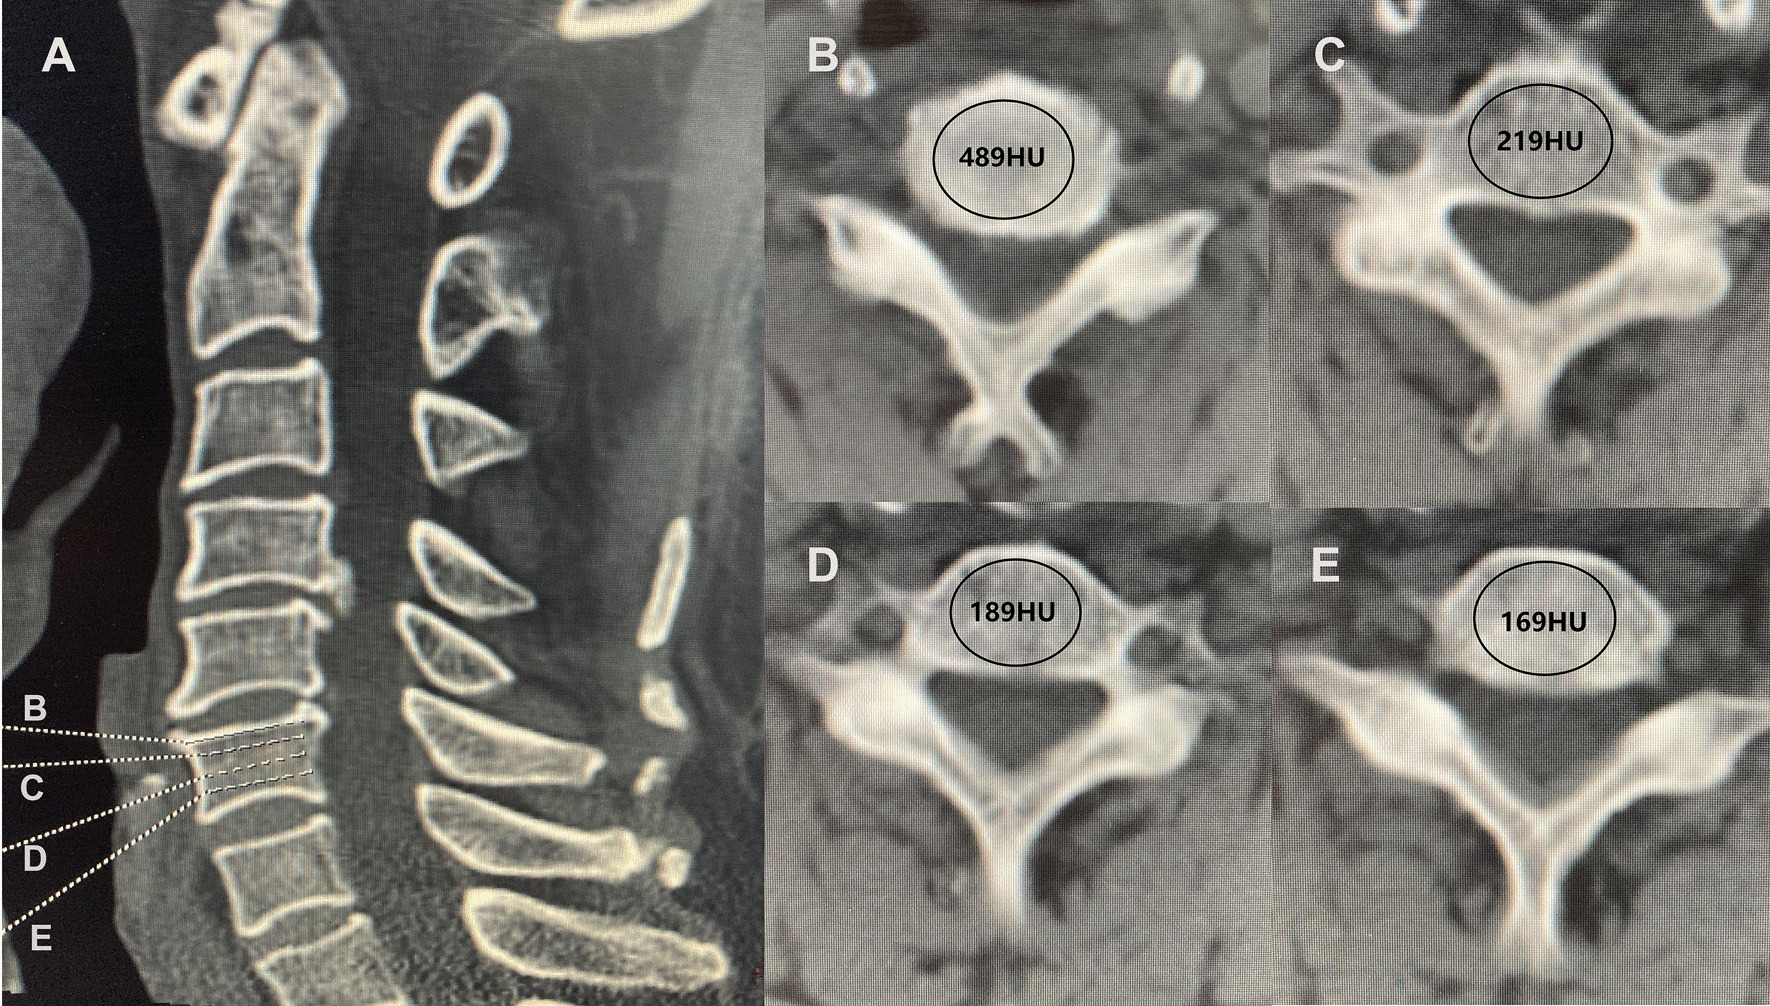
Mid-sagittal (A) and axial CT images demonstrating the measurement of endplate (B) and vertebral body (C–E) HU value
